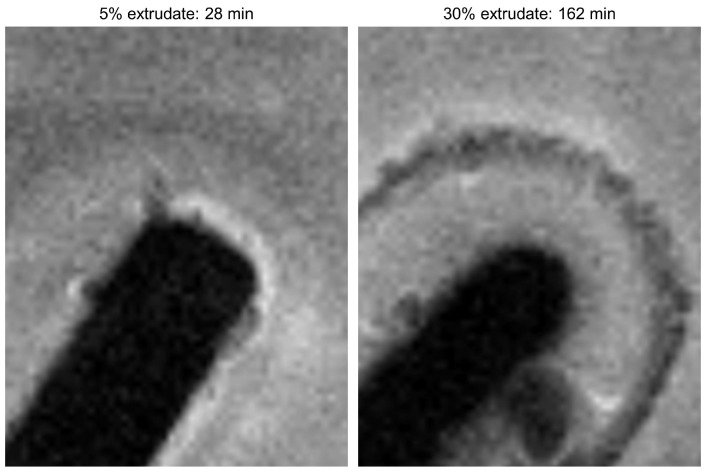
Zoom of the images collected for the 5% extrudate (28 min) and 30% extrudate (162 min) to highlight the well-defined region of intermediate MRI contrast present in the 30% extrudate.
